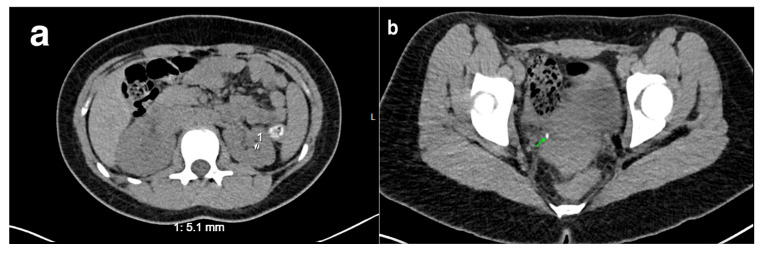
Nephrolithiasis in a 16-year-old female (indicated by number 1). (a) CT reveals a left intrarenal stone. (b) CT highlights a stone at the right ureterovesical junction (indicated by the green arrow).
